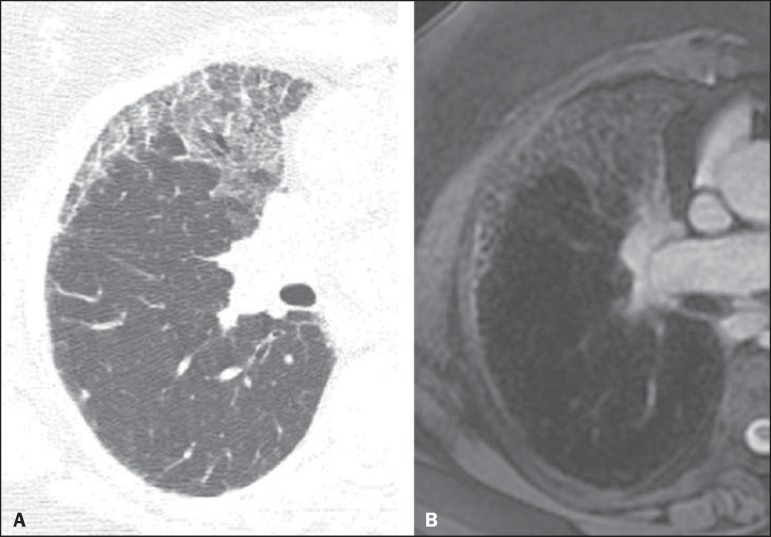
A: Axial HRCT image showing homogeneous, segmental
